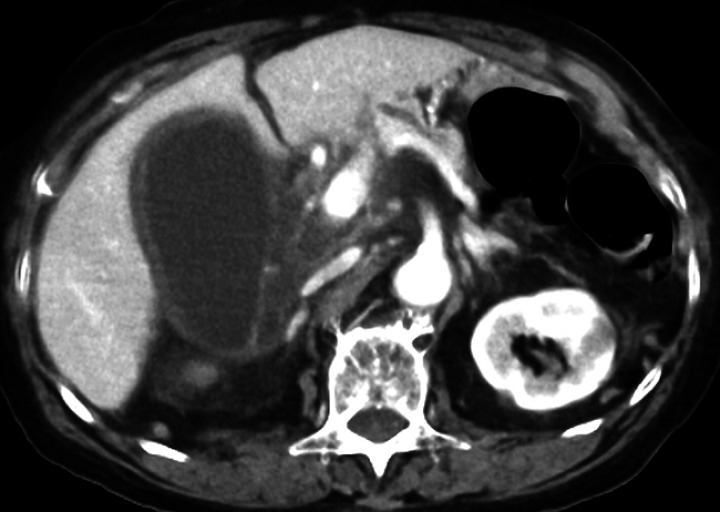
Computed tomography showing swelling of the gallbladder and thickening of the gallbladder wall.
